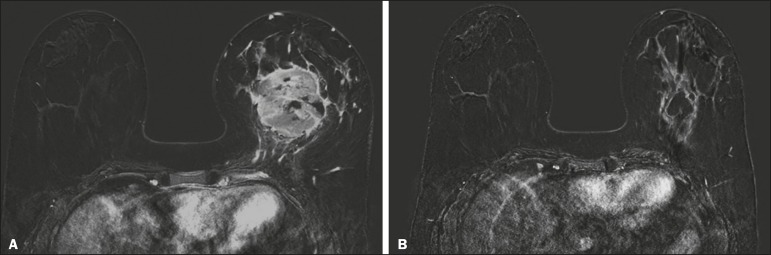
Example of a false-negative case. Pre- and post-NAC MRI (A
